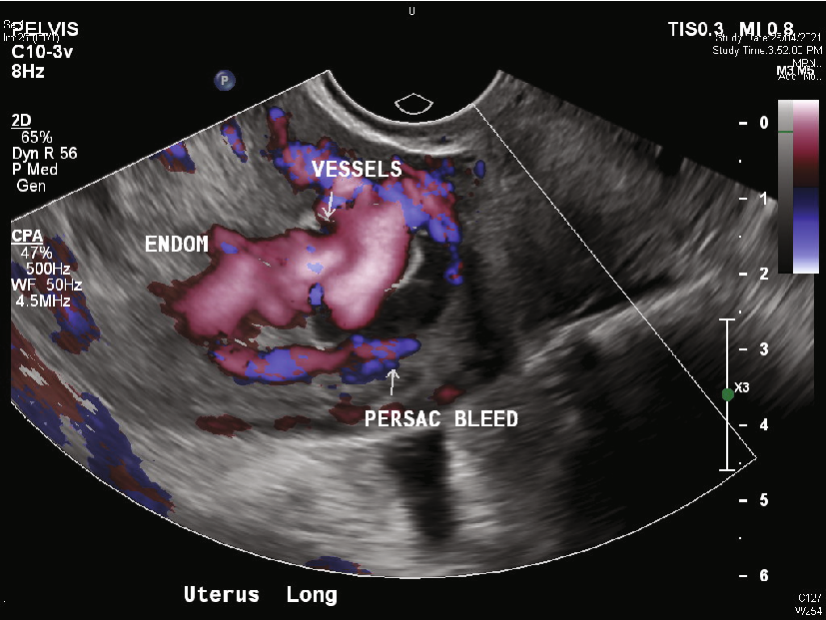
Transvaginal ultrasound suspicious for a uterine AVM.
